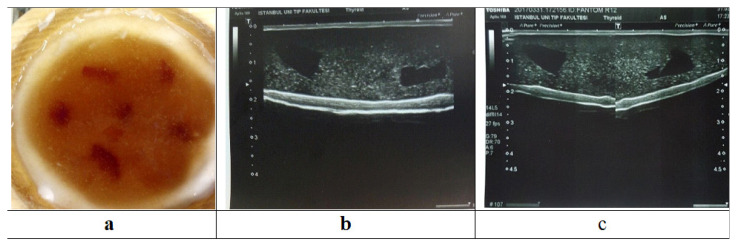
a) Image of top view of SH-P6 before coating with PDMS, b) ultrasonography images of 2 CTs in one frame and c) individual CT obtained from two different frames.
